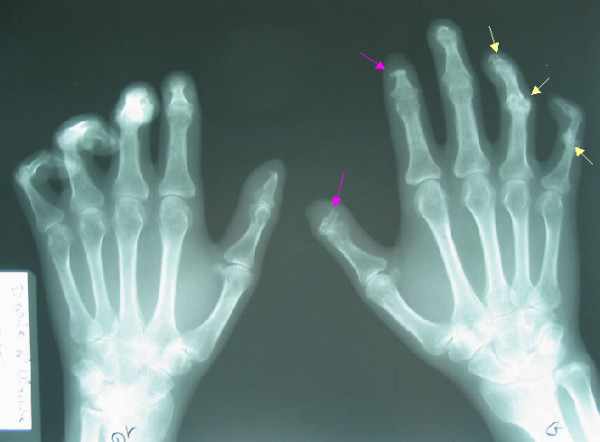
X-ray of hands showing. - Erosion of the fourth and the fifth proximal inter phalangeal joint on the left hand. - Terminal phalangeal tuft resorption (arrow).
